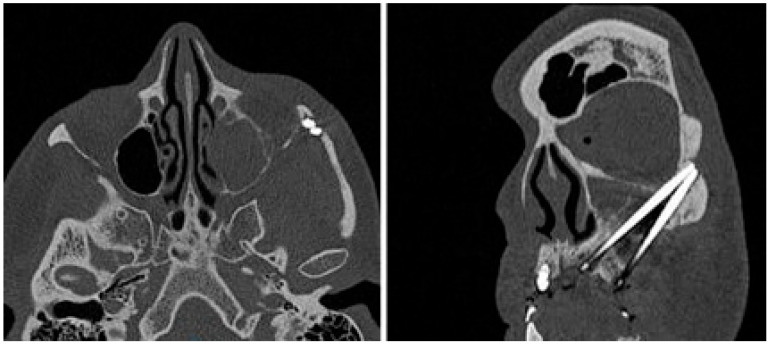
CT axial and coronal view: two ZIs on the left zygoma with apex in contact without adequate inter-implant distance and outer cortical bone resorption.
